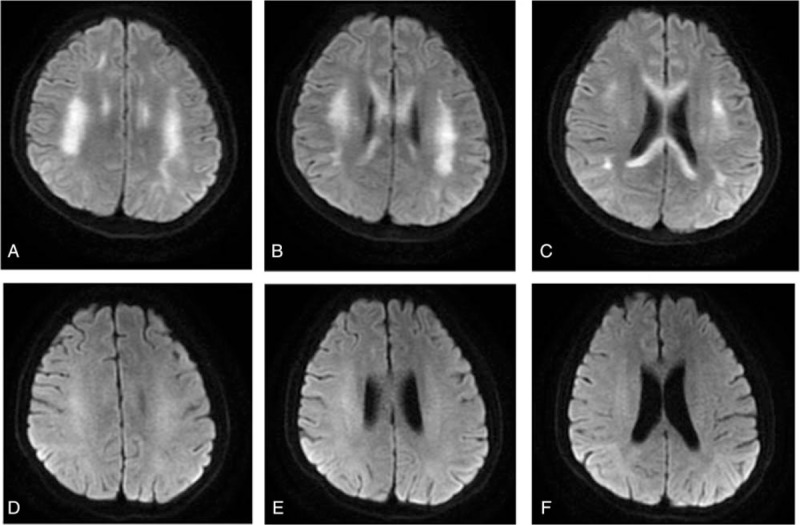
Brain magnetic resonance imaging before and after treatment. (A–C) High signal of white-matter (the corpus callosum, bilateral centrum semiovale, periventricular area) and symmetrical subcortical high signal lesions on diffusion-weighted imaging before treatment. (D–F) High signal lesions on the original MRI disappeared after treatment.
